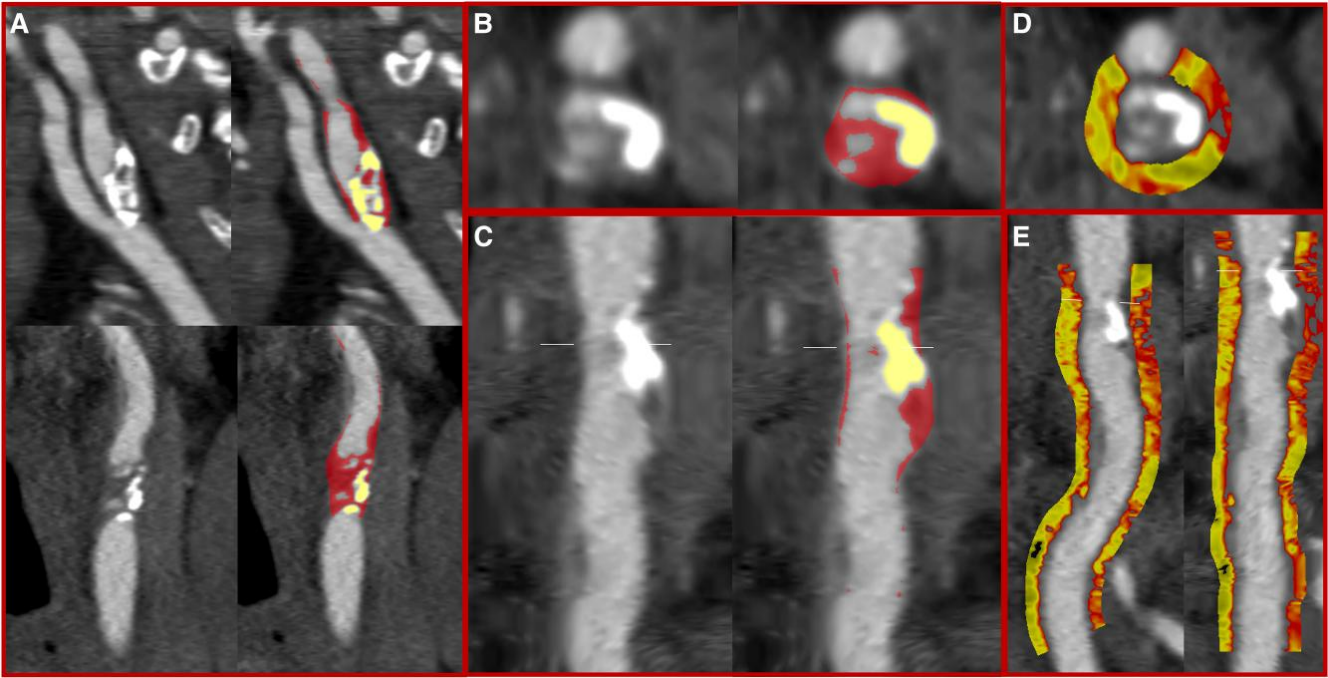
Quantitative plaque analysis. Sixty-six-year-old patient presenting with a left sided transient ischaemic attack and a 60–65% stenosis in the left internal carotid artery on Doppler ultrasound, who proceeded to carotid endarterectomy. CT showed a severe stenosis in the proximal left internal carotid artery with a high burden of calcified and non-calcified plaque on curved planar reformations (*A*), cross-sectional images (*B*), and straightened curved planar reformations (*C*). Quantitative plaque analysis shows non-calcified plaque in red (*A–C*), calcified plaque in yellow (*A–C*), and peri-vascular adipose tissue attenuation in yellow/orange (*D* and *E*).
